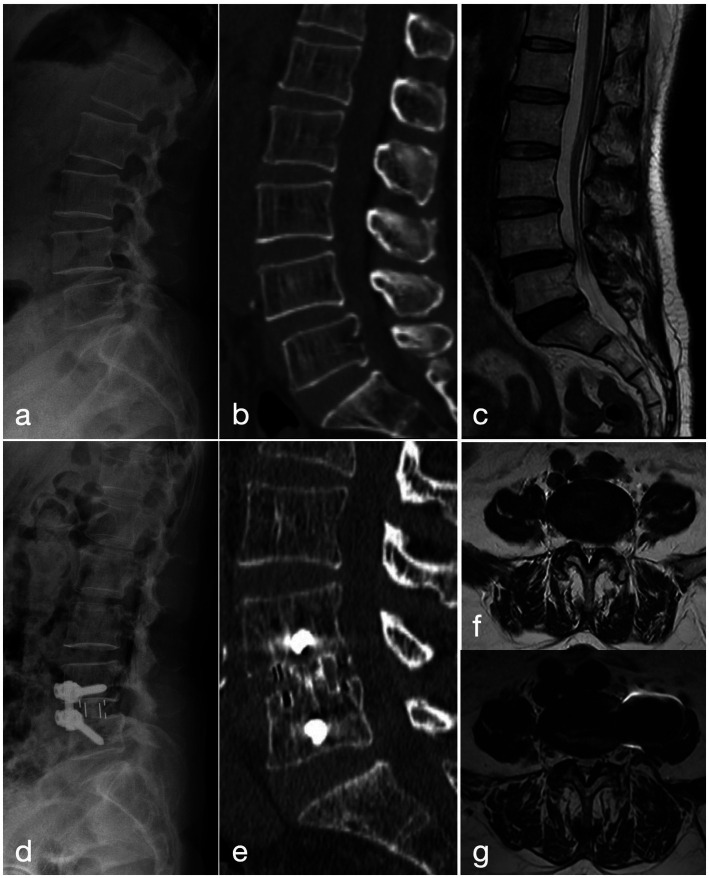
A 56-year-old woman who had been suffering from neurogenic claudication for 1 year, with symptoms worsening over the last 3 months, was treated with the OLIF-AF procedure. (a–c) Pre-operative lateral radiograph, CT and MRI show grade-I anterior spondylolisthesis of L4. (d) Post-operative lateral radiograph demonstrates well-positioned instrumentation and interbody cage with good reduction of the slip. (e) CT at 2 years confirms solid interbody fusion. (f) Pre-operative MRI reveals severe L4/5 central canal stenosis. (g) MRI at 2 years shows increased canal volume and positive nerve-root sedimentation sign.
